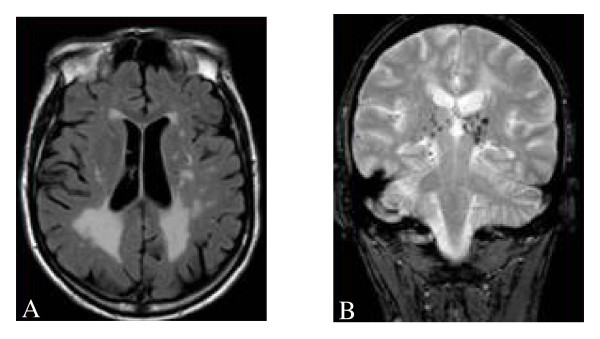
Cerebral white matter hyperintensities, lacuna and microbleeds: A. Fluid-attenuated inversion recovery (FLAIR)-weighted axial MRI section showing multiple white matter lesions in the cerebral hemispheres in a 53-year-old male patient who had a Fazekas score of 9. B. Lacuna and microbleeds in the same patient. Courtesy: Dr Robert CARLIER and Dr Frédéric COLAS, CHU Raymond Poincaré, Garches, France.
